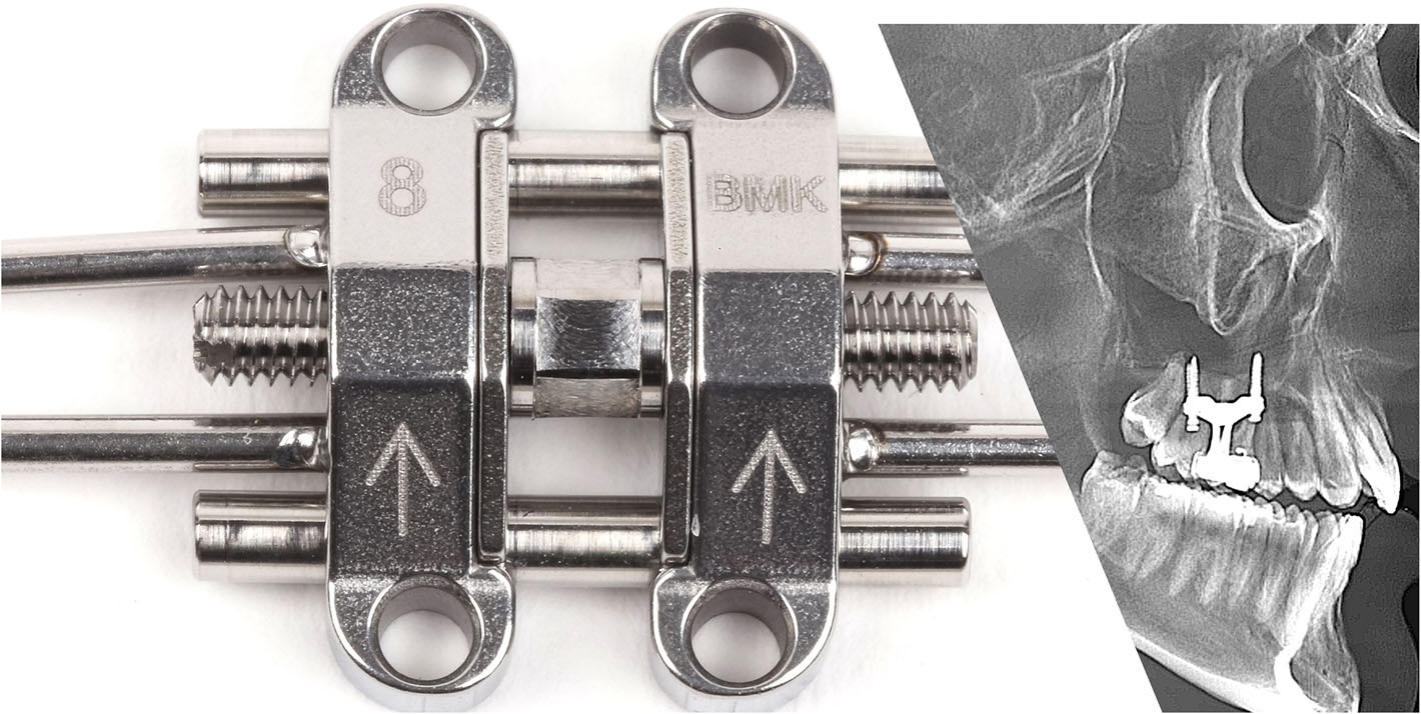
Midfacial skeletal expander device and X-ray showing bicortical engagement of the four microimplants
